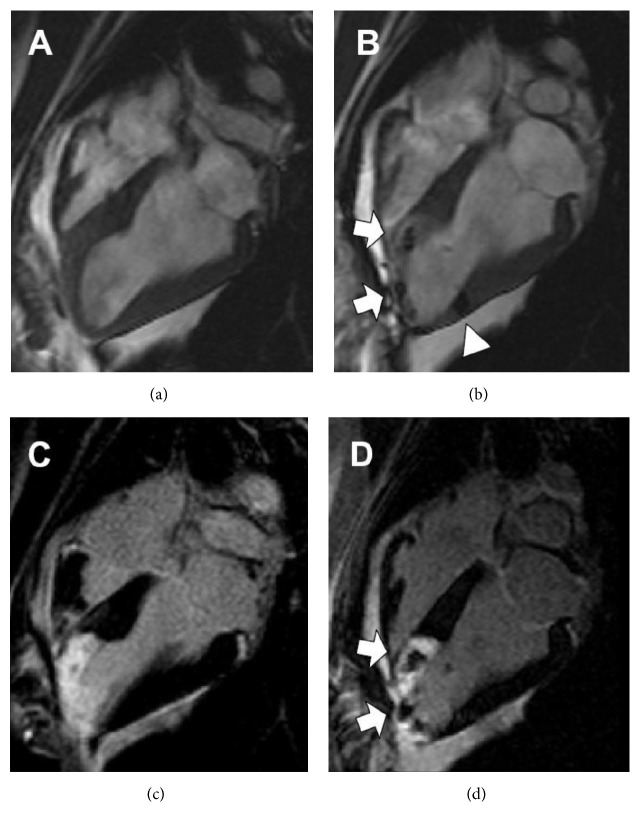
In vivo* visualization immediately before and after IFP-MSC injection. Long-axis SSFP MRI view of left ventricle before (a) and after (b) transcatheter injection of 4 × 106 IFP-labeled MSCs into the infarct at the apex (arrows) and adjoining normal myocardium (arrowhead). Delayed enhancement MRI highlights areas of nonviable infarcted myocardium using the same view as above, before (c) and after (d) injection of IFP-labeled MSCs. MSCs appear dark against hyperenhanced infarct (reproduced with permission [27]).
